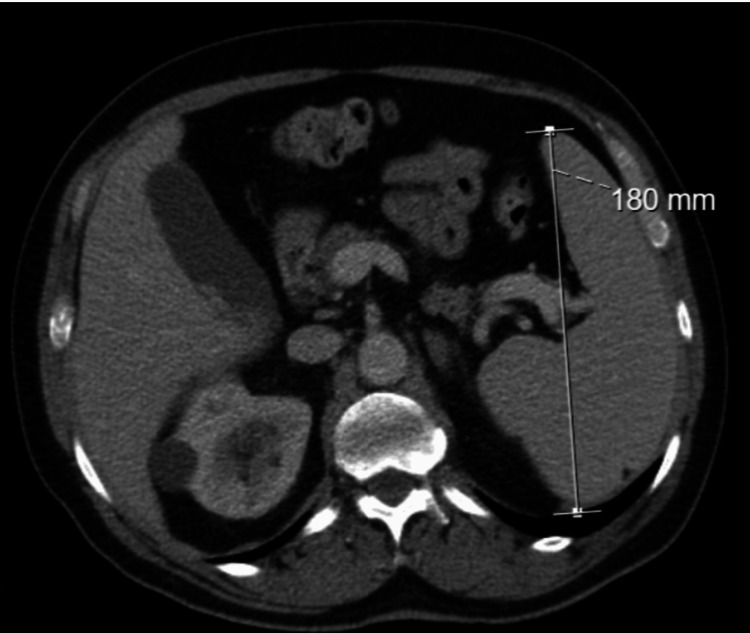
Abdominal computed tomography showing splenomegaly (~18 cm) without focal splenic lesions or associated lymphadenopathy.
